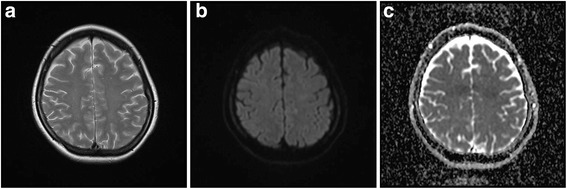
Magnetic resonance imaging study 7 weeks postoperatively shows resolution of all the lesions in T2-weighted (a), diffusion-weighted (b), and apparent diffusion coefficient map images (c)
